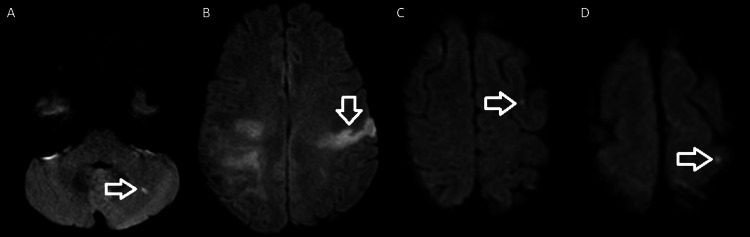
New DWI hyperintensities (arrow) on (A) the left cerebellum, (B) the left precentral gyrus and parietal, and (C, D) the left high frontal
